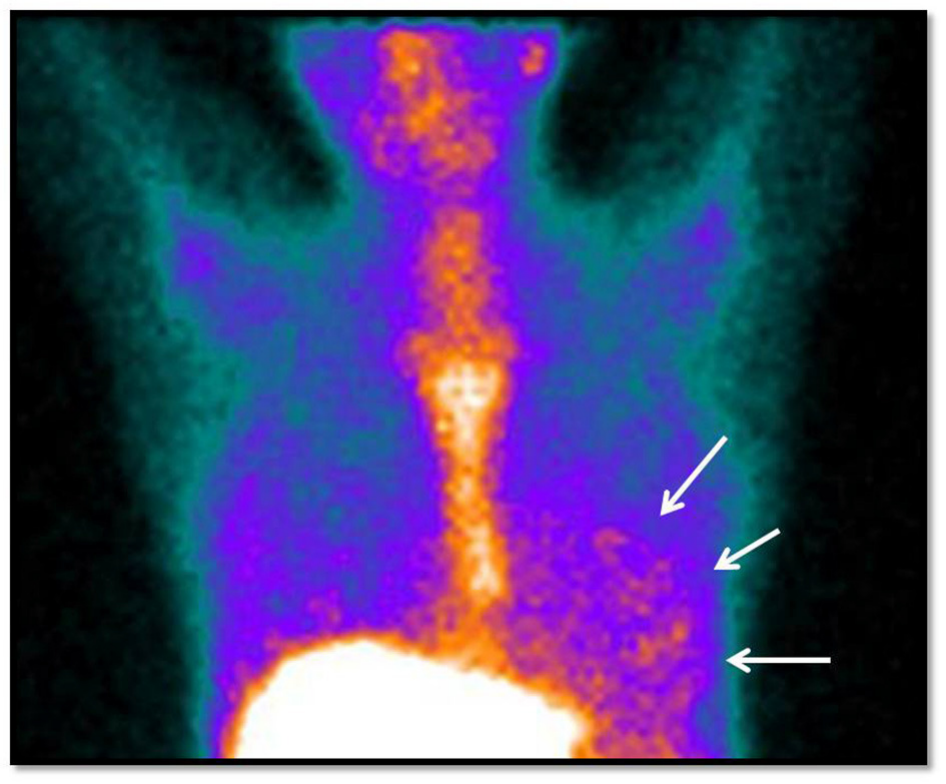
Positive gallium-67 cardiac scintigraphy (anterior view of the thorax). Image obtained 72 h after radiopharmaceutical administration, showing diffuse and mild gallium-67 uptake in cardiac projection (arrows), indicating an inflammatory myocardial process. Physiological gallium-67 uptake was observed in the liver and bones (sternum and ribs).
